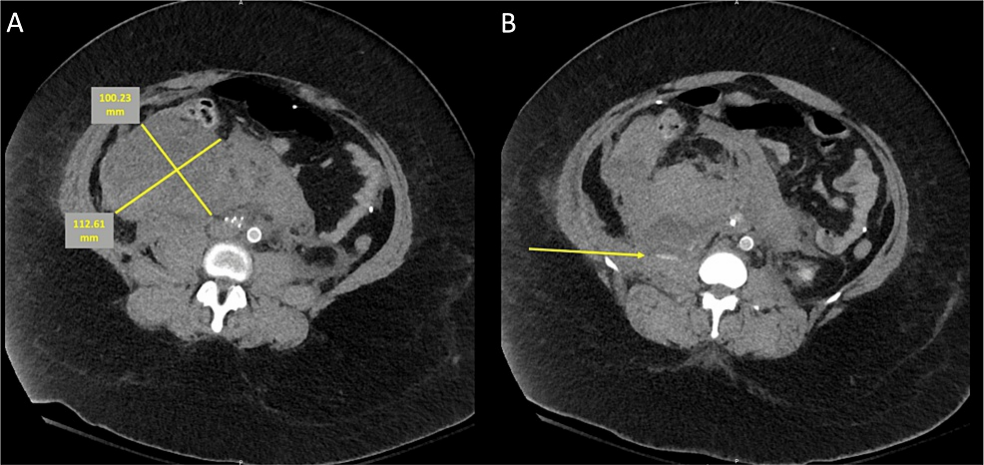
CT post-IVC filter placement demonstrating hematoma formation (A) and extravasation of blood from the lumbar branch (B) IVC - inferior vena cava
